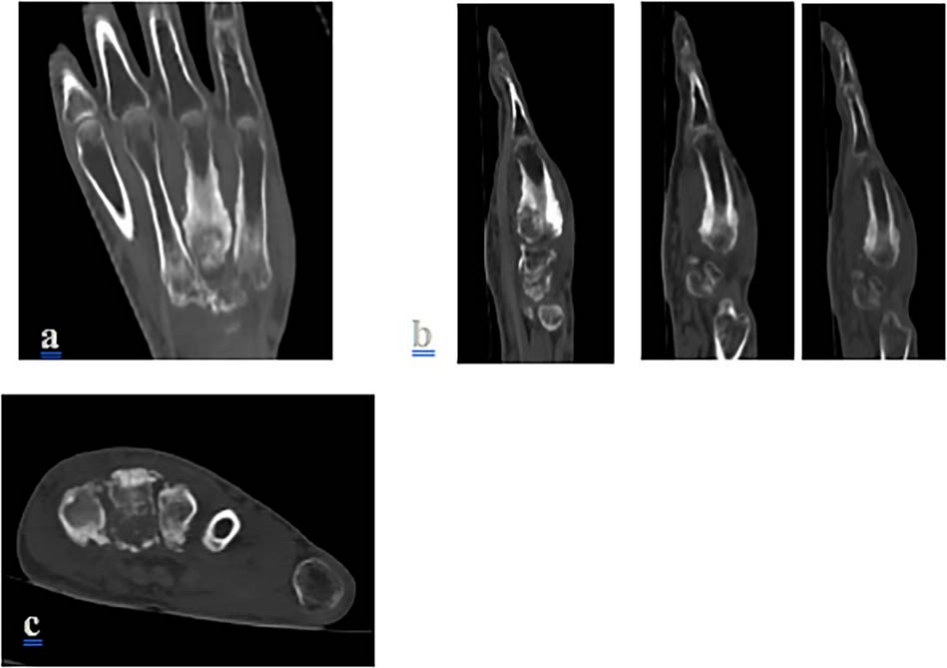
CT reconstruction of the coronal (a), sagittal (b) and axial image (c) showing cortical hyperostosis and sclerosis of the 3rd, 2nd and 5th metacarpal bones.
